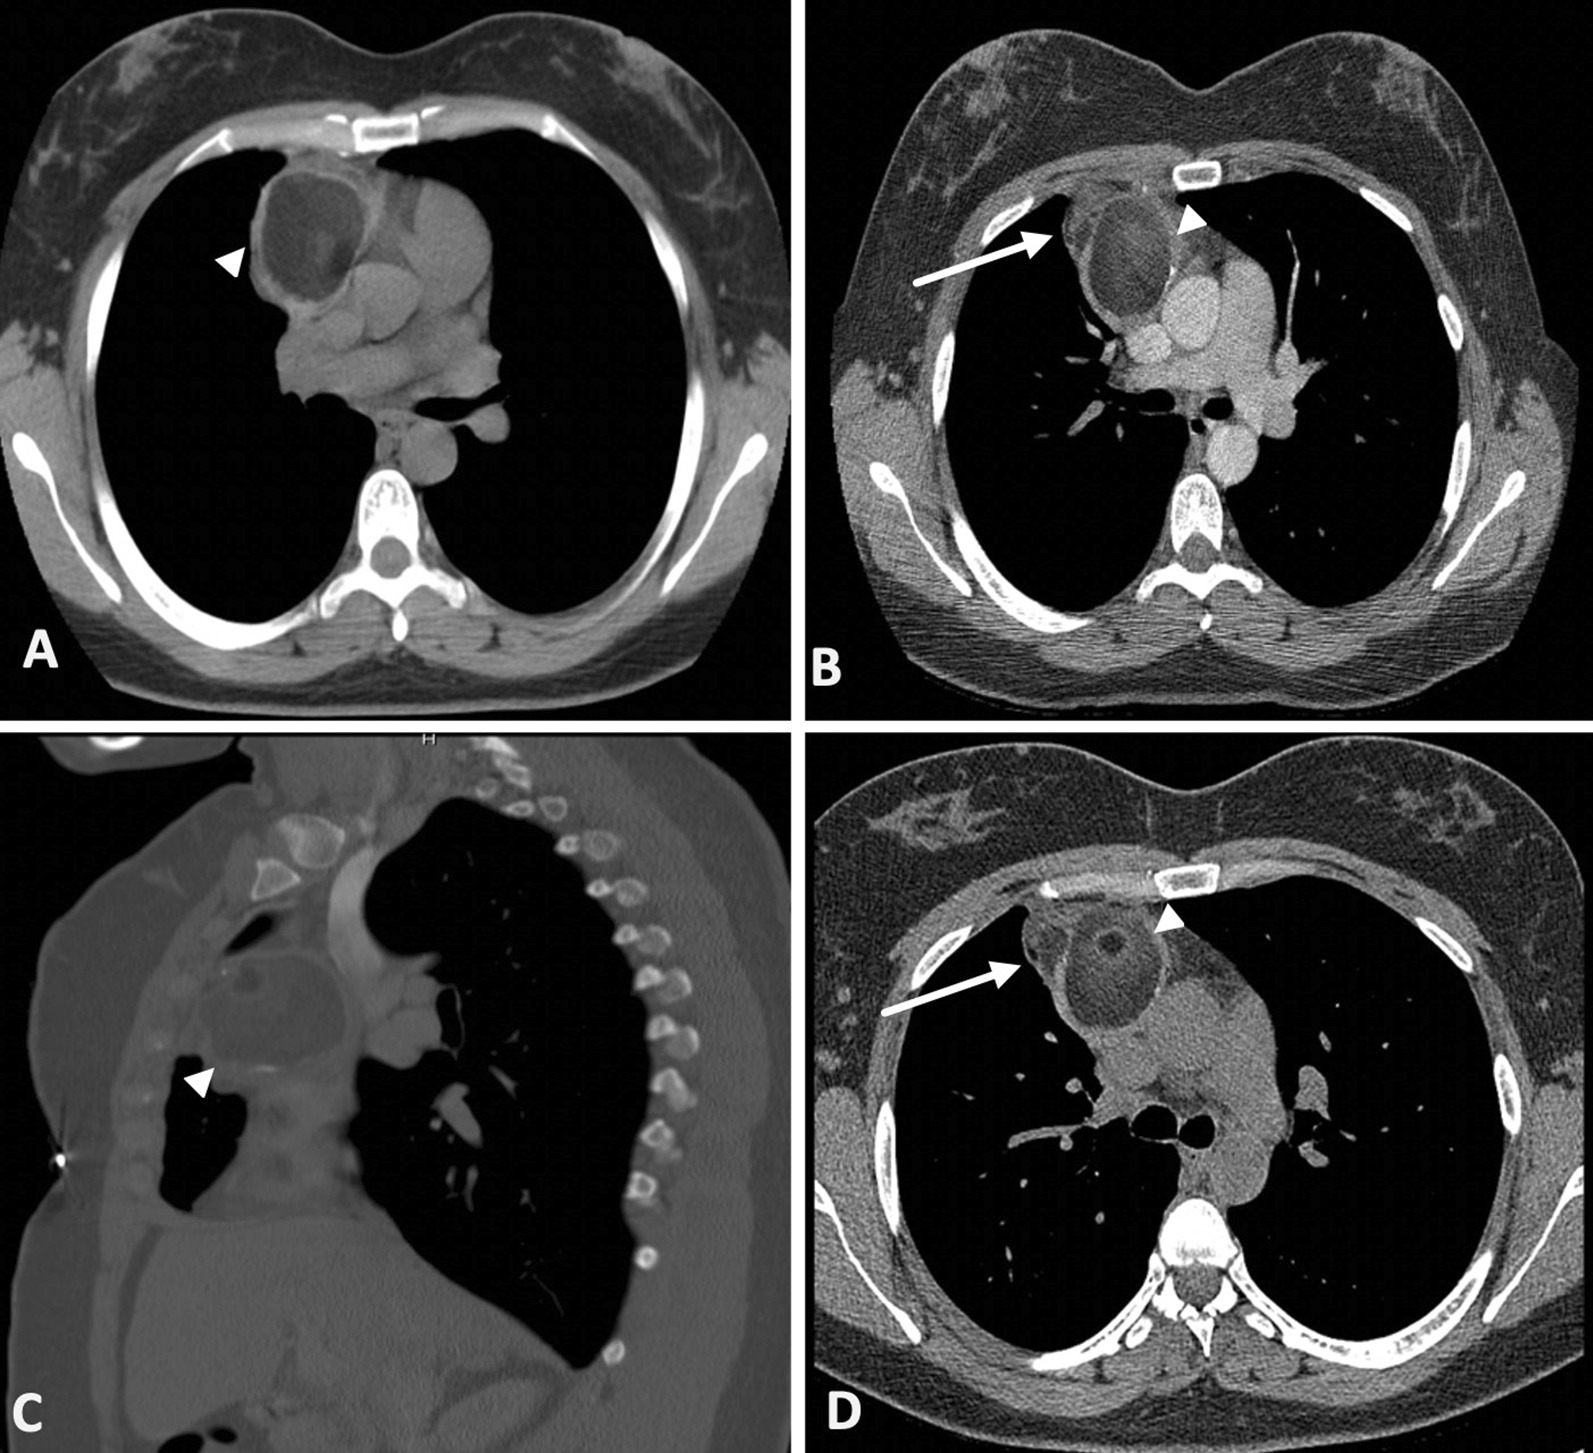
Initial axial computerized tomography (CT) of the thorax in 2014 showing mediastinal mass (A). Axial (B) and sagittal (C) CT of the thorax in 2018 showing increase in size of heterogenous mass with a new lobulation (arrow). Axial CT thorax prior to resection (D) showing an increase in size of the new lobulation (arrow)
